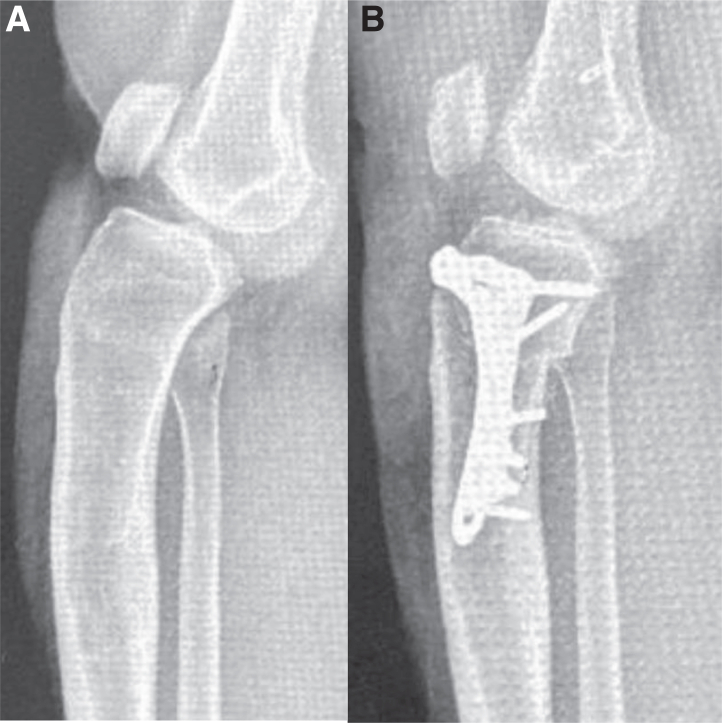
Lateral radiographs of the right knee. (A) A preoperative lateral radiograph of the right knee shows excessive proximal tibial sagittal slope with associated severe proximal tibial anterior subluxation and patella baja. (B) A 3-month postoperative lateral radiograph of the right knee shows large improvement of the sagittal proximal tibial slope, less anterior subluxation of the proximal tibia, and improved patellar height.
